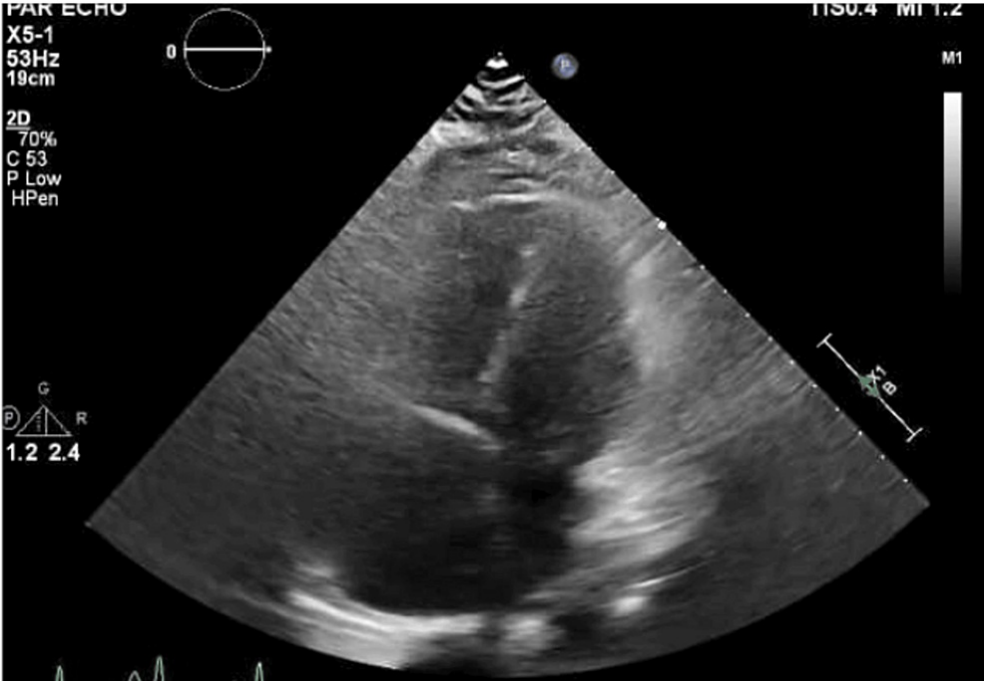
Transthoracic Echocardiogram Showing Apical Four-Chamber View With Dilated Right Ventricle and Right Atrium
